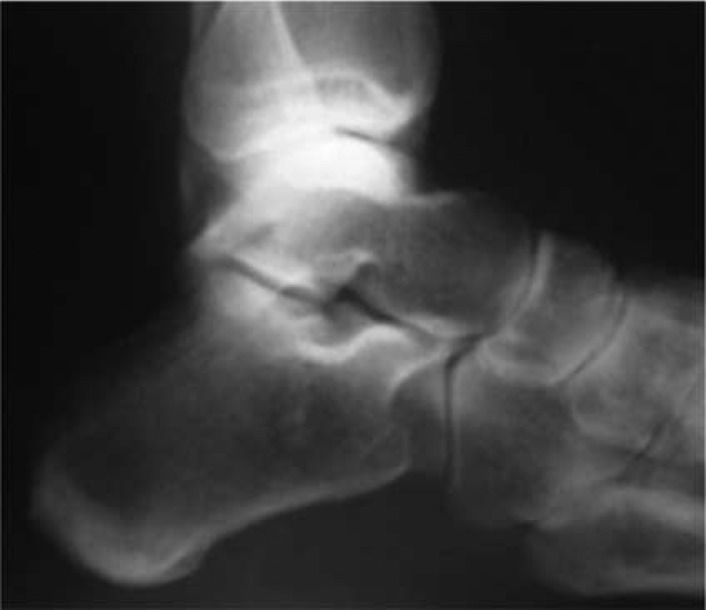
Plain lateral radiographic view without evidence of changes in shape and skeletal structure.
